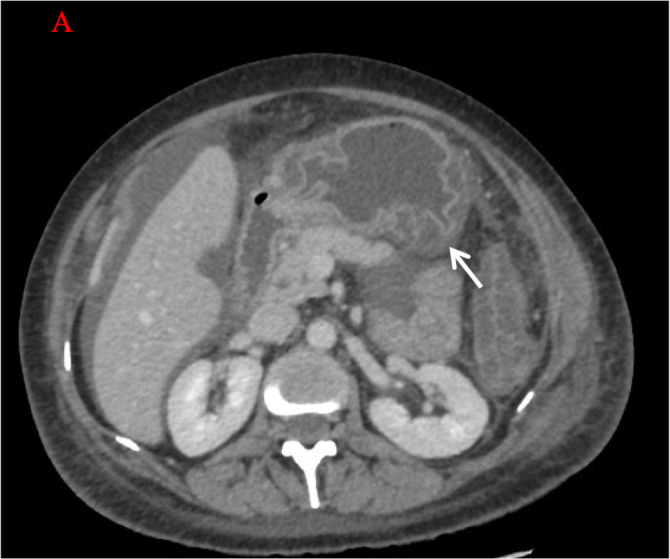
Contrast-enhanced CT of the abdomen images in axial (A) and coronal (B) plane demonstrating stomach wall oedema (arrow) and ascites.
